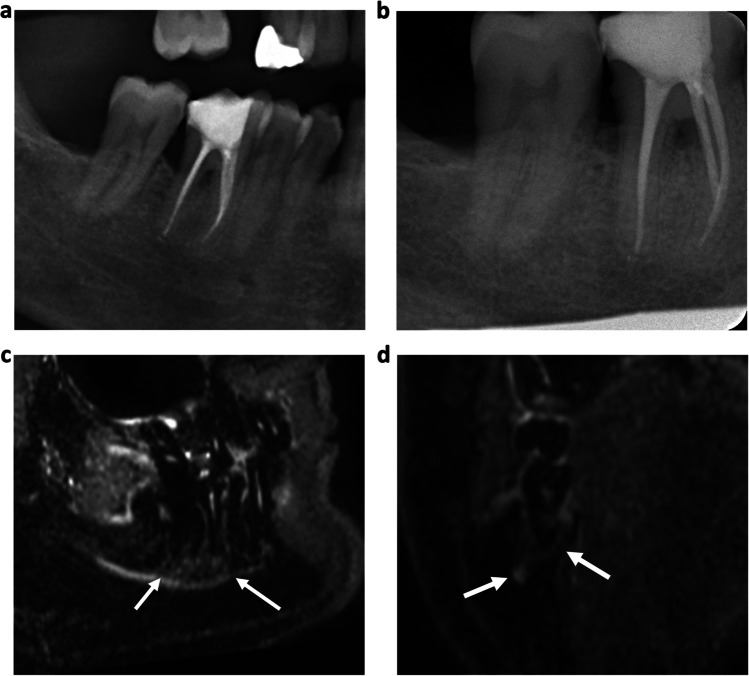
64-year-old patient with known periodontitis. a OPT and b dental radiograph of the tooth 46 after root canal treatment showing no distinct radiolucency. c Sagittal and d coronal reconstruction of a 3D STIR sequence showing a bright edema periapical around the root of the treated tooth. The alveolar bone edema on MRI (white arrows) indicates an inflammatory process but might be also associated with physiological changes. Therefore, findings have to be evaluated together with the results from patient anamnesis and clinical examination
